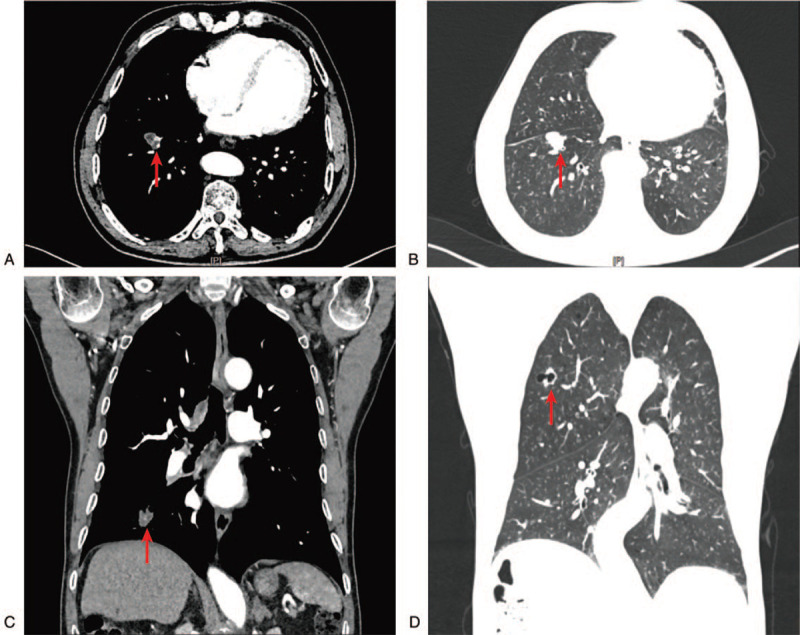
Chest CT. A-C, In the right lower lobe (S_8_), 1 nodule measured 20 × 15 × 16 mm showed clear boundary, lobulated margin, and multiple hive-like low density areas in the center (arrows). D, In the right upper lobe (S_1_), another nodule measured 20 × 14 × 13 mm showed irregular margin, uneven thickness of cavity wall, and local nodular solid density shadow (arrow). CT = computed tomography.
